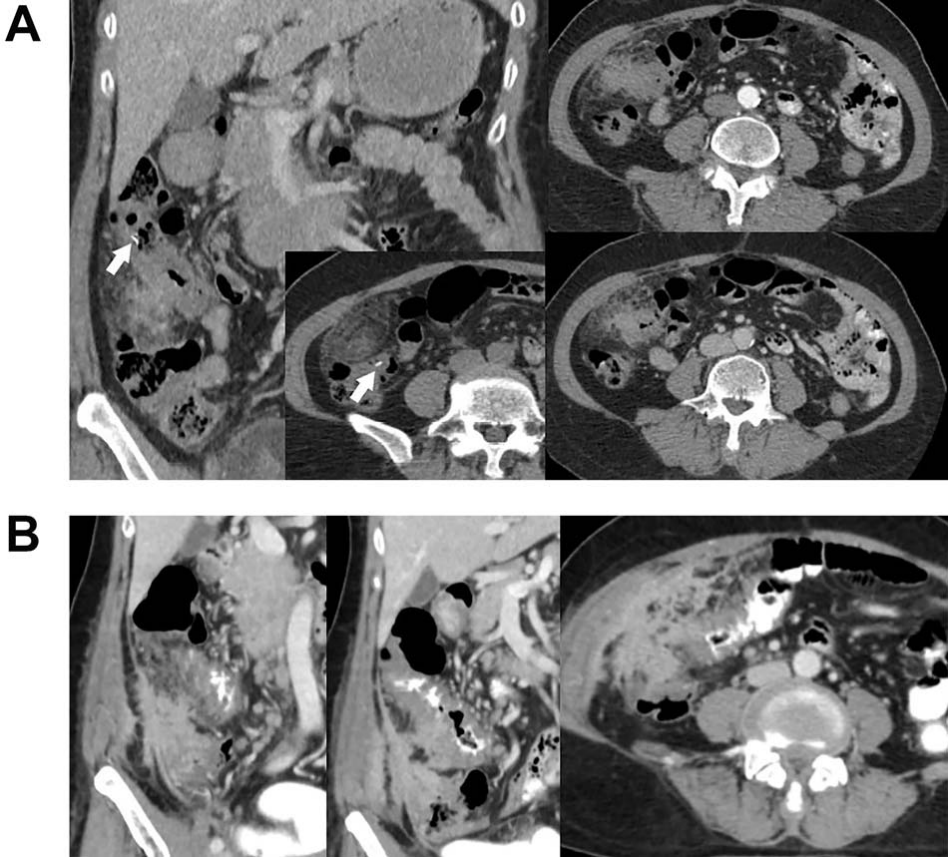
Contrast-enhanced abdominal CT. Images (A) and (B) were obtained 5 months apart. Image A shows irregular circumferential thickening of the colonic hepatic flexure. An elongated foreign body is also visible in the area (arrow). Image B shows an increase in the size and extension of the thickening. There is also a newly-detected heterogenous enhancing mass-like lesion involving the lateral wall of the cecum and proximal ascending colon, with enhancement indicating irregular thickening of the adjacent peritoneum.
